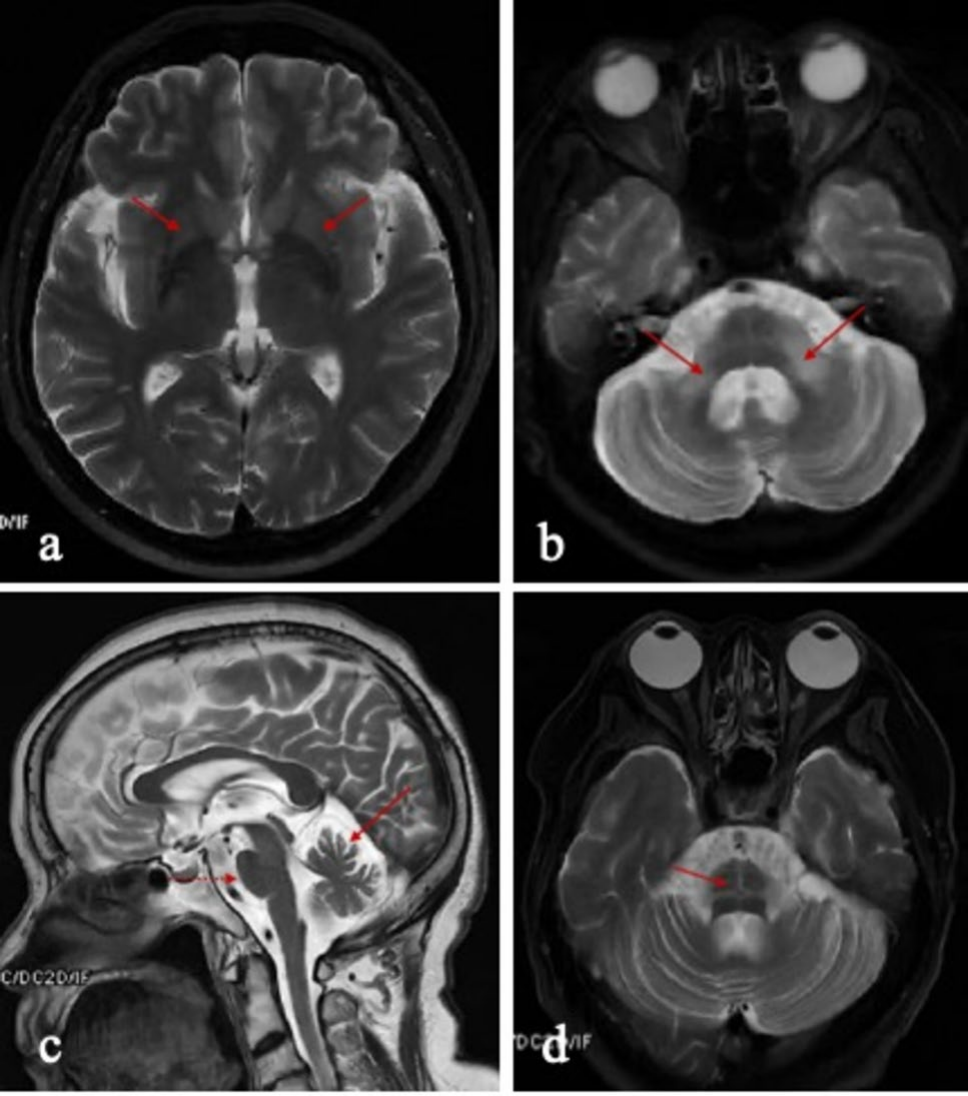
MRI brain imaging in MSA patients. a Demonstrates putaminal atrophy (red arrows). b Atrophy and hyper-intensity signal of middle cerebellar peduncles (red arrows). c Pontine atrophy (red dashed arrow) and cerebellar atrophy (red solid arrow). d “Hot cross bun” sign (red arrow)
